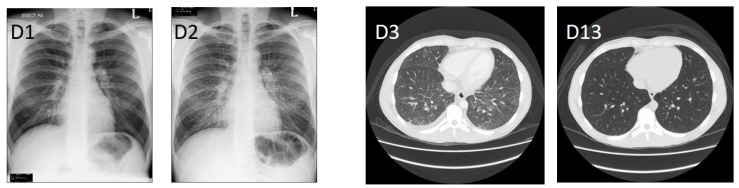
Chest X-rays upon admission (D1) and subsequent day (D2). Contrast-enhanced CT scan after oral and intravenous contrast administration at the day of hypotensive shock (D3), and post-treatment (D13).
